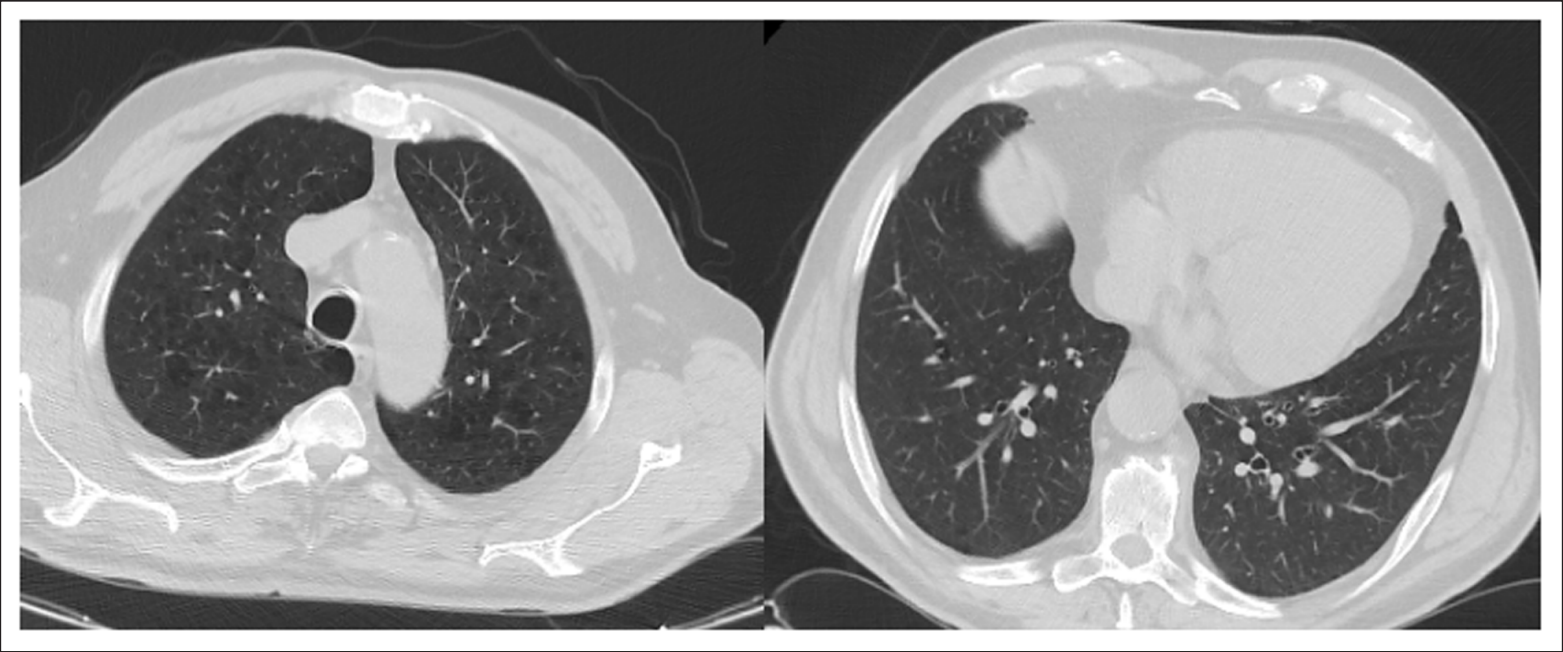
RT-PCR +, CO-RADS 1: This 65-year-old male patient had hypertension, cardiovascular disease, chronic kidney disease, and hyperlipidemia. He presented to the emergency department with shortness of breath and cough. He had hypoxemia. Laboratory parameters compatible with COVID-19 were d-dimer: 990 ng/mL, ferritin: 510 ng/mL, fibrinogen: 443 mg/dL. The patient with positive RT-PCR test and emphysematous changes in both upper lobes of the lungs on CT was hospitalized with the diagnosis of COVID-19. It was evaluated as CO-RADS 1. The patient recovered and was discharged on the 7th day.
